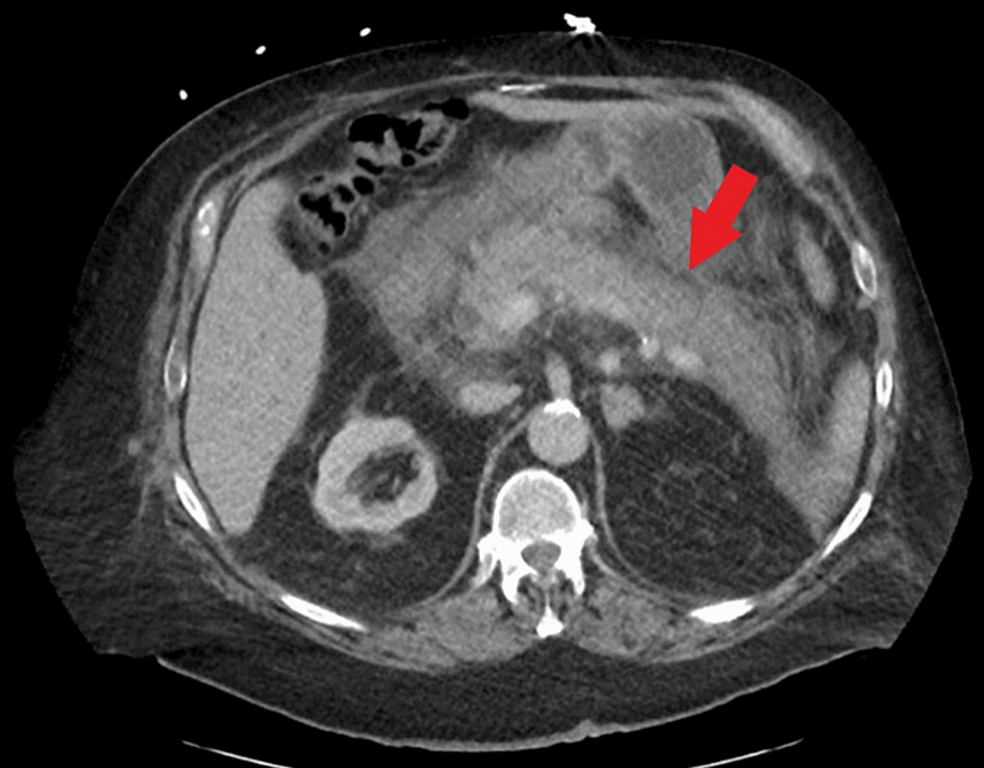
CT of the abdomen and pelvis with contrast revealing extensive peripancreatic inflammatory changes, extending to the adjacent pylorus and duodenum suggestive of moderate-to-severe pancreatitis. There is also notable intrahepatic and extrahepatic biliary ductal dilation.
